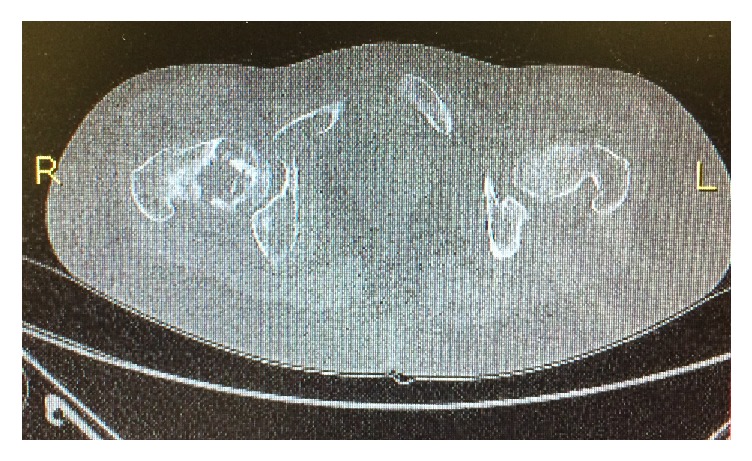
Computed tomography at age 17. Osteolytic lesions and areas of “ground-glass” opacity in the right femur.
